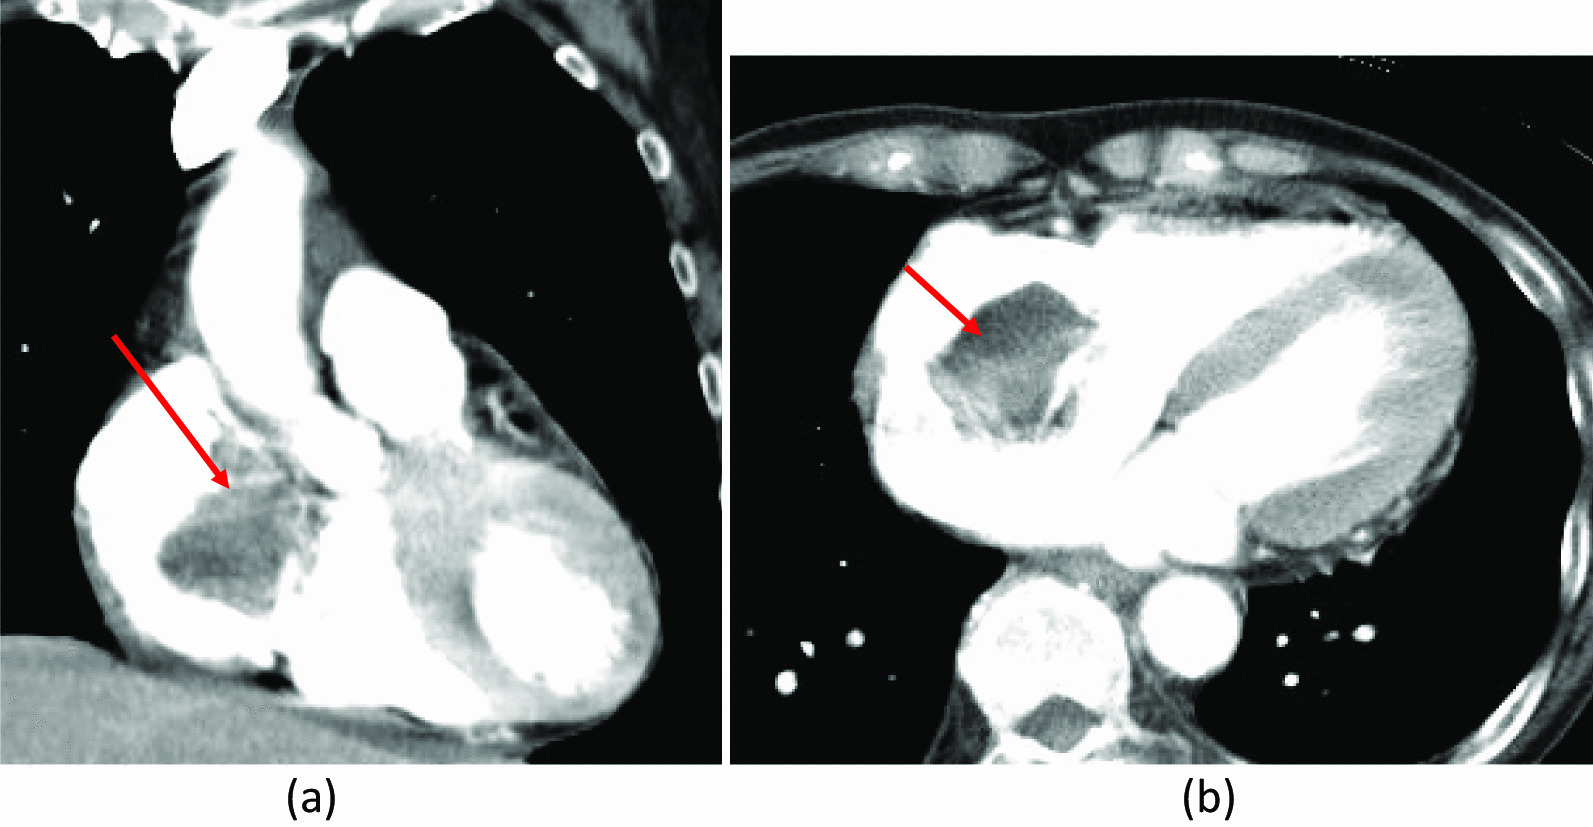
Enhanced computed tomography revealing a large defect in the right atrium (arrows). a Coronal view. b Axial view
